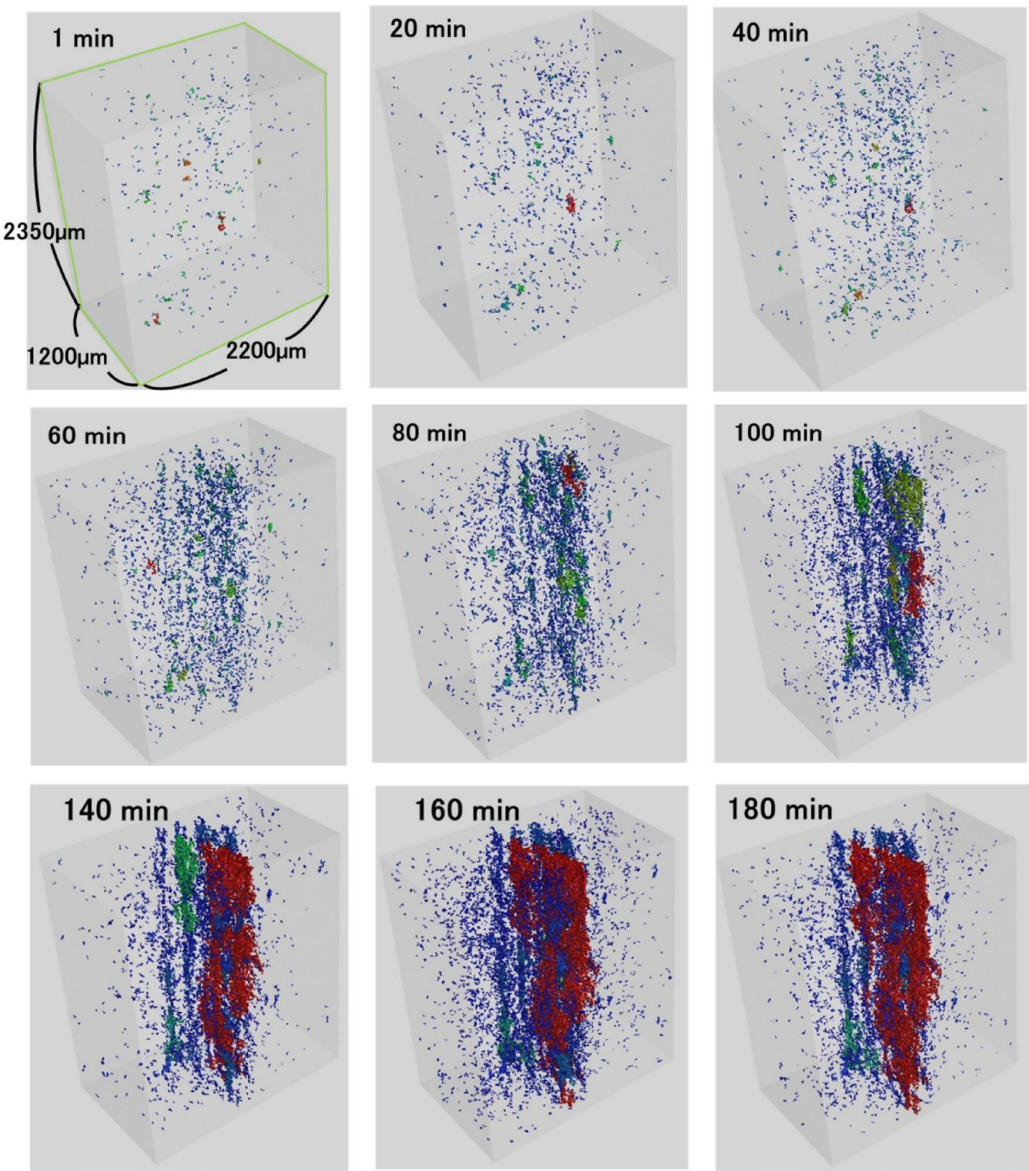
The course of three-dimensional X ray CT image of a gel sample during drying process in the 0–180 min.
